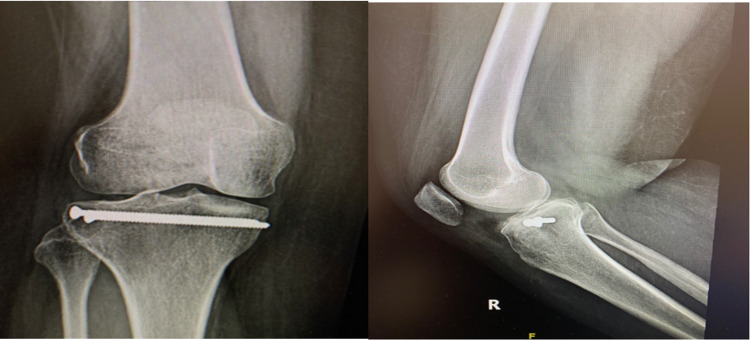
Anteroposterior and lateral X-ray showing healed fracture without any evidence of displacement or subsidence.
